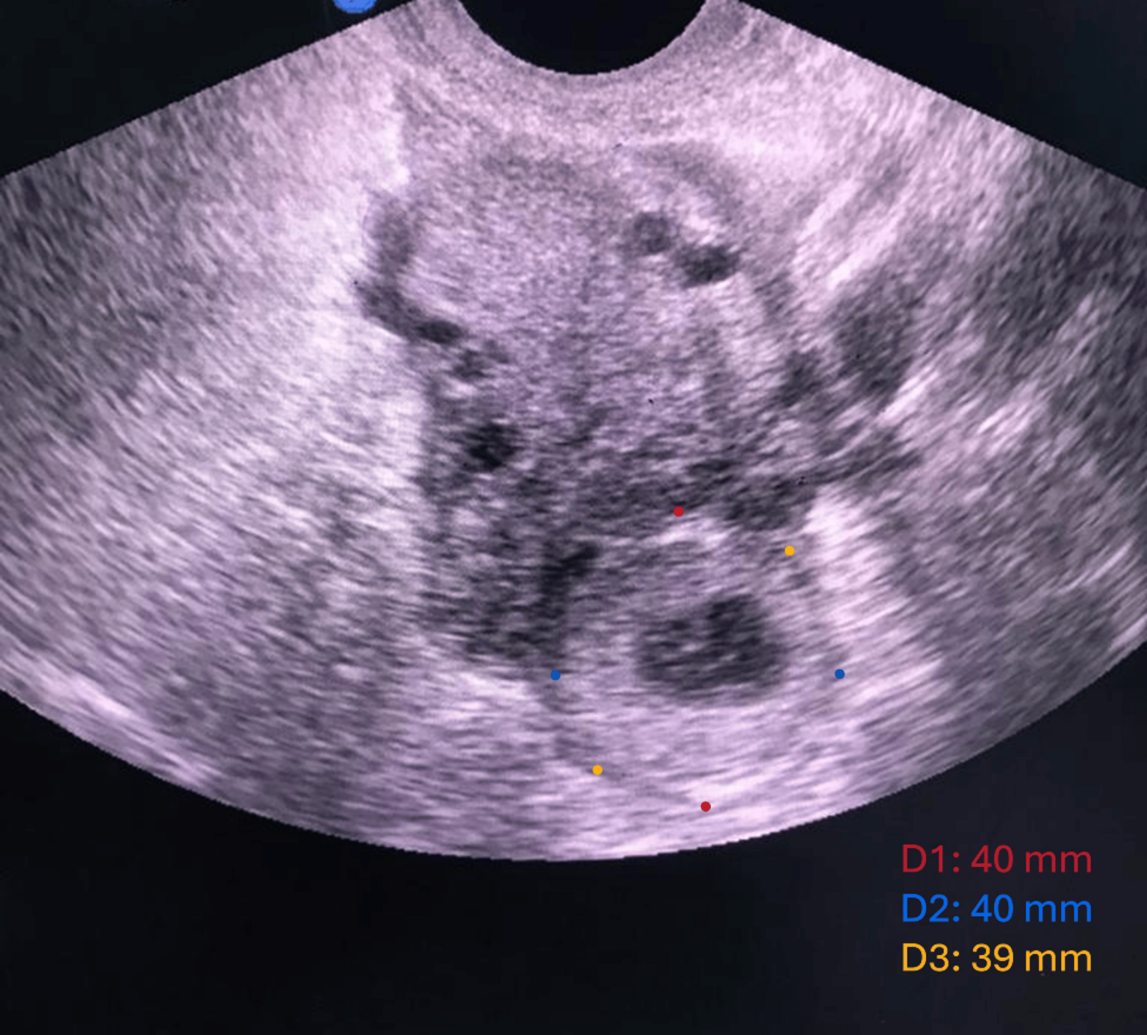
Transvaginal ultrasound shows right adnexal mass.
